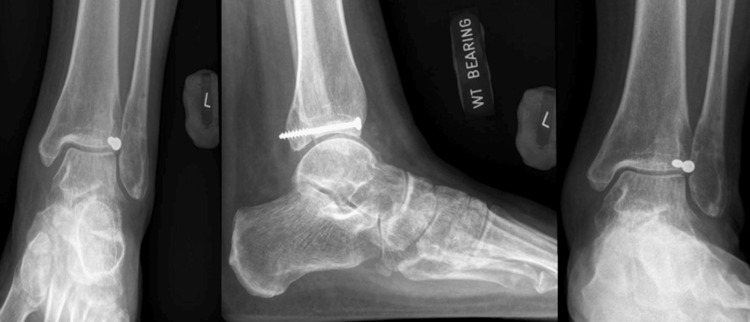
Final left ankle X-ray anterioposterior/lateral/mortise views
